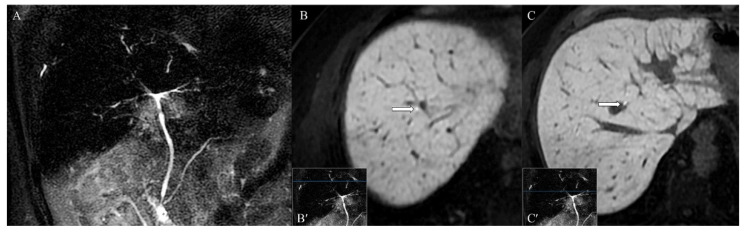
(A) MRI cholangiography phase, in the absence of anamnesis, does not allow a confident differential diagnosis with primary sclerosing cholangitis. (B,C) After administration of Gd-EOB-DTPA, hepatobiliary phase images (B) above and (C) below the stenosis demonstrate physiological opacification of the intrahepatic bile ducts and, despite the stenosis, physiological contrast flow.
